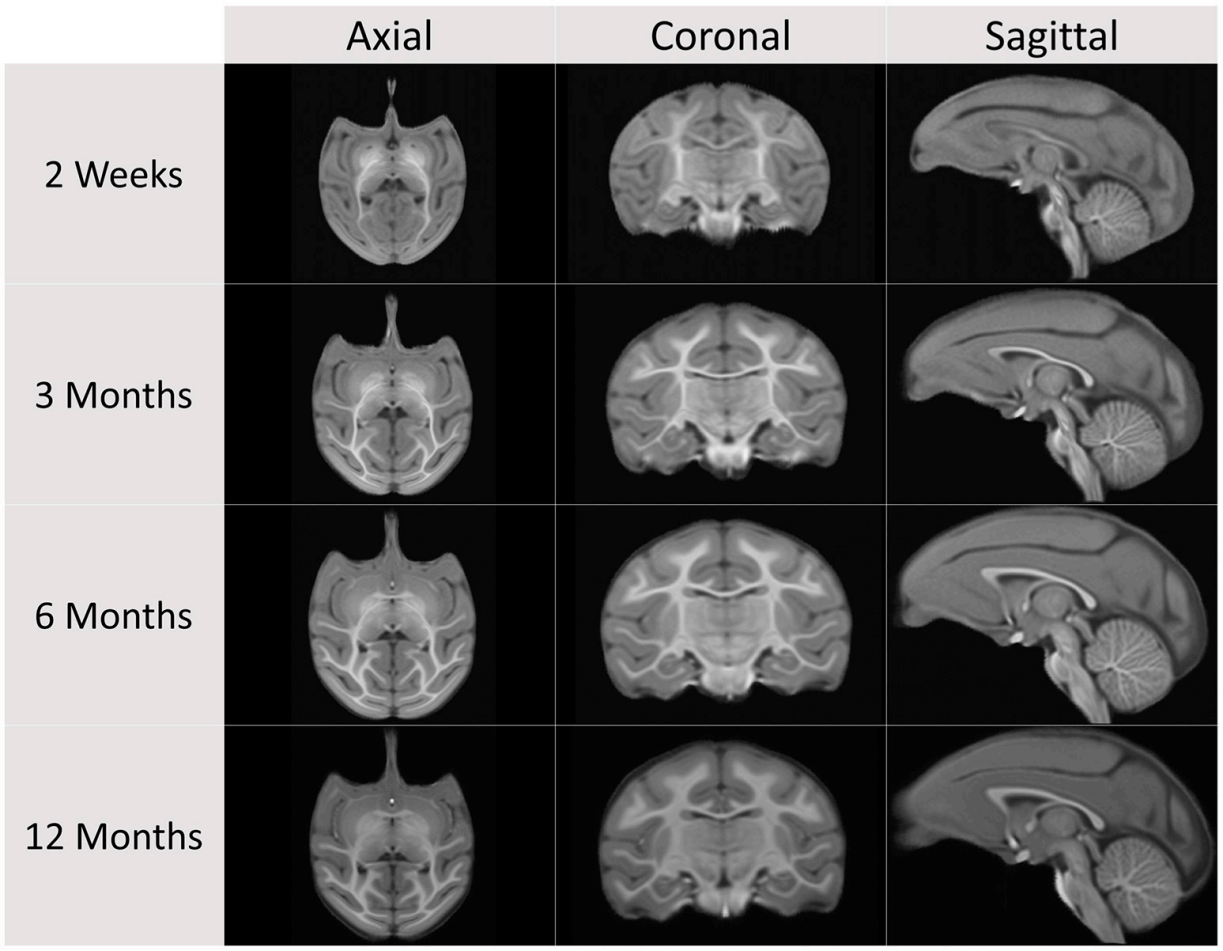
Cross-sectional average T1-weighted atlas images at the four atlas building ages.
